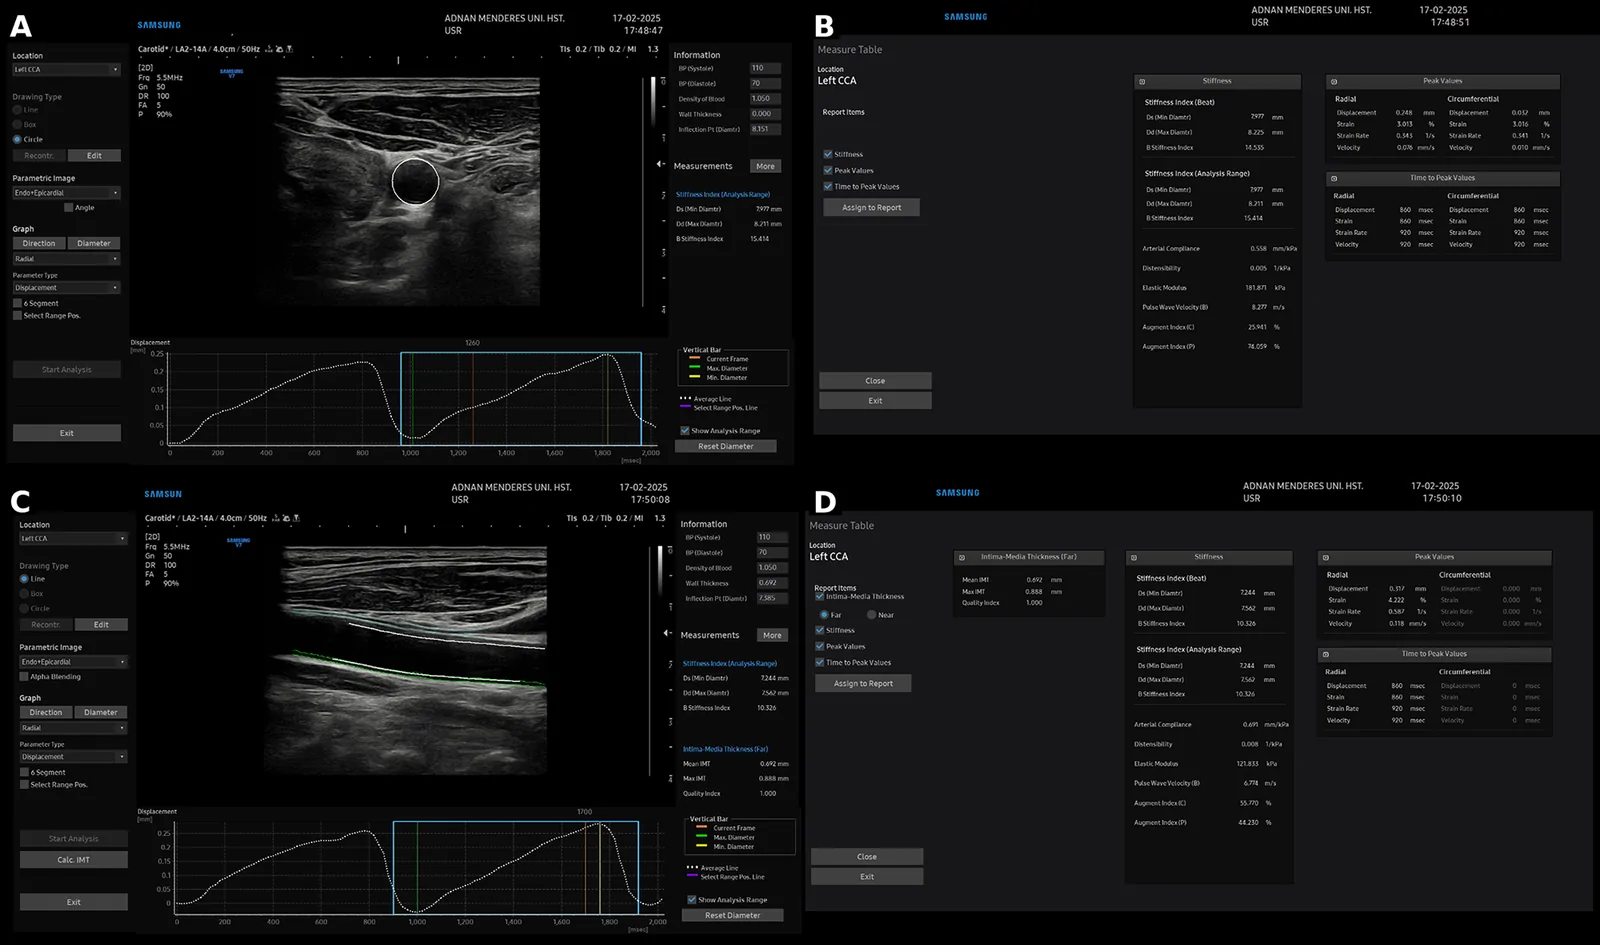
Representative STCS-US examination in a participant with metabolic syndrome. (A) Transverse-plane carotid ultrasound image with speckle-tracking analysis. (B) Transverse-plane stiffness and strain measurements generated by the Arterial Analysis software program. (C) Longitudinal-plane carotid ultrasound image with speckle-tracking analysis. (D) Longitudinal-plane stiffness and strain measurements generated by the Arterial Analysis software program
